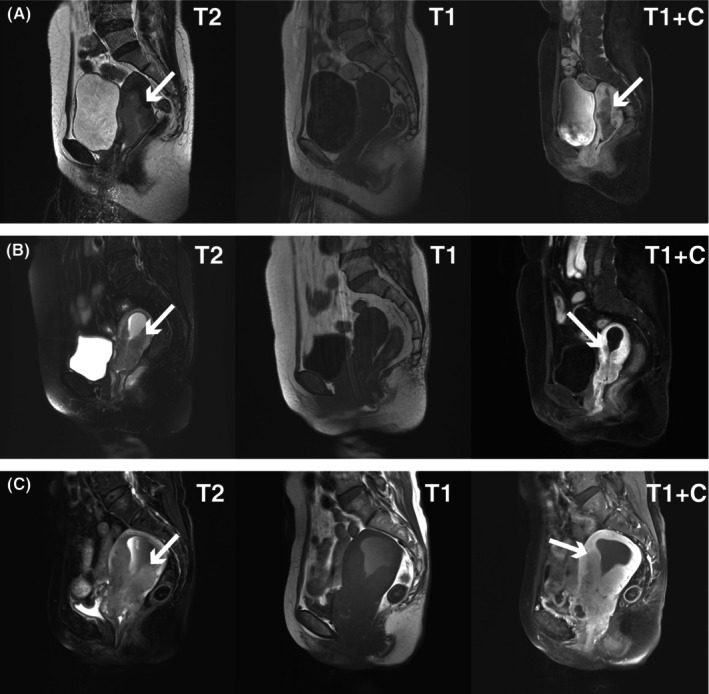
Pelvic MRI images showing uterine body invasion of the cervical cancer patients. (A–C) Uterine body invasion (white sick arrows) shown in the pelvic MRI images of three cervical cancer patients, with an intermediate‐intensity signal on sagittal T2‐weighted images, low‐intensity signal on sagittal T1‐weighted images, and intermediate reinforcing signal on sagittal T1‐enhanced images.
